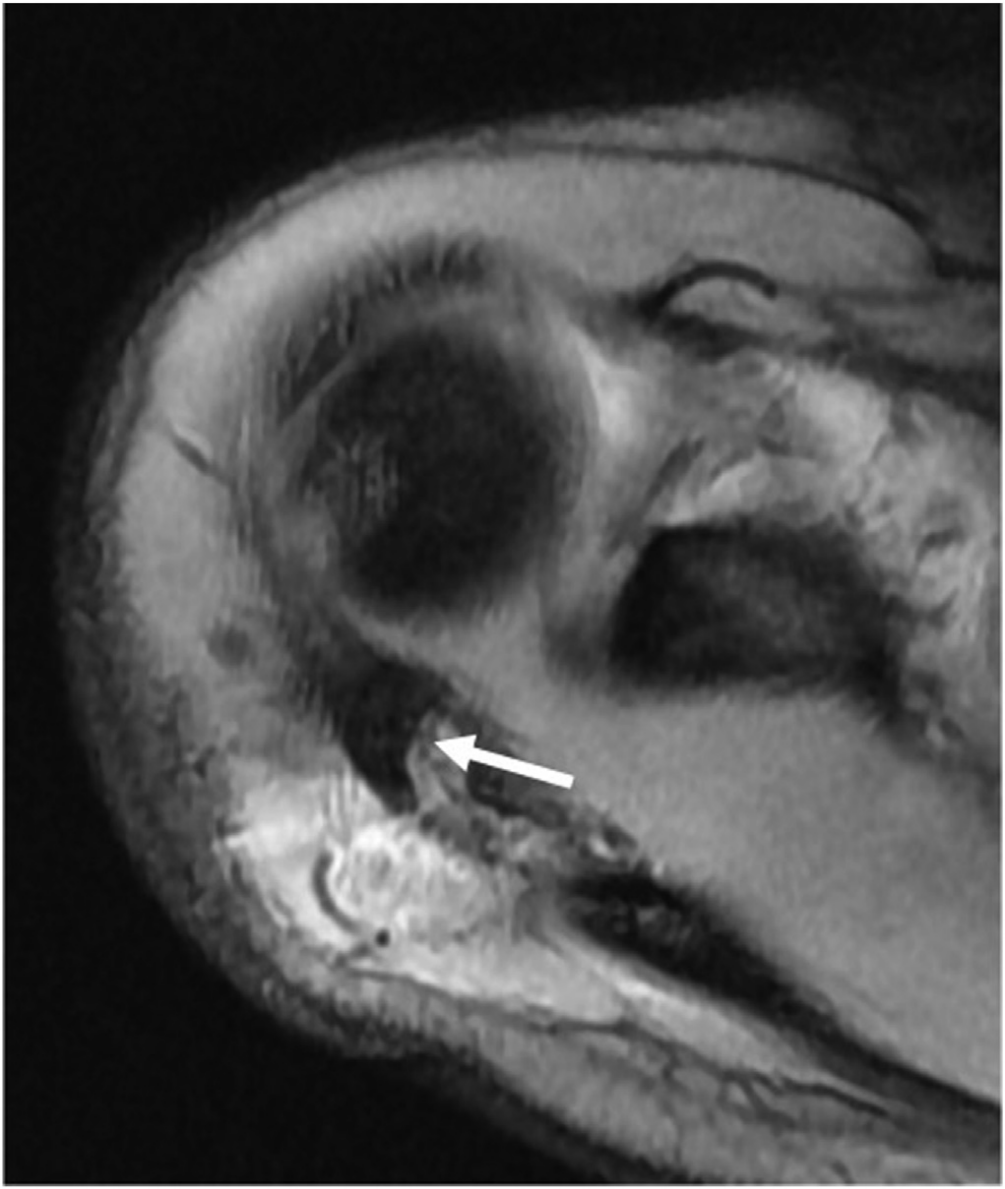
Preoperative axial plane MRI showing a displaced meso-acromion ossification center with a posteriorly curved physis (arrow). *MRI*, magnetic resonance imaging.
